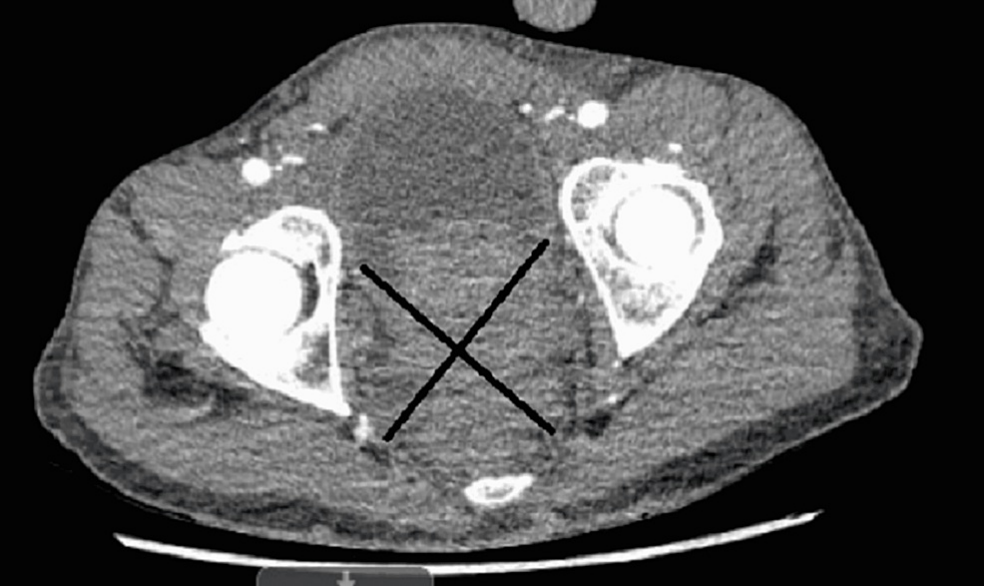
A computed tomography scan of the pelvis revealed prostatic enlargement with a distended bladder
